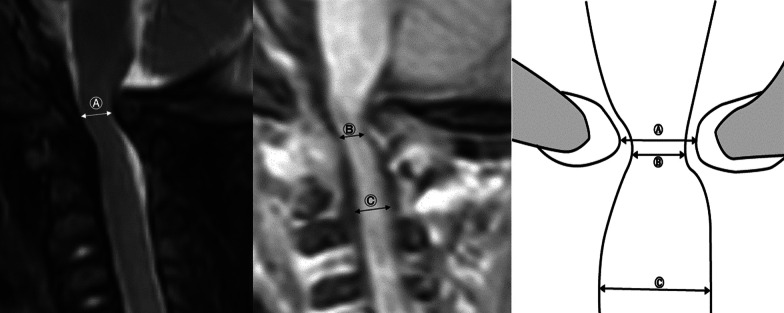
‘FM AP’ (A) was measured using T2 sagittal MRI images. The T1 sagittal MRI image was used to measure ‘Cord thickness narrowest’ (B) and ‘Cord thickness C2’ (C). We created a new parameter called ‘Cord constriction ratio’ and calculated it as ‘1—(B/C)’
